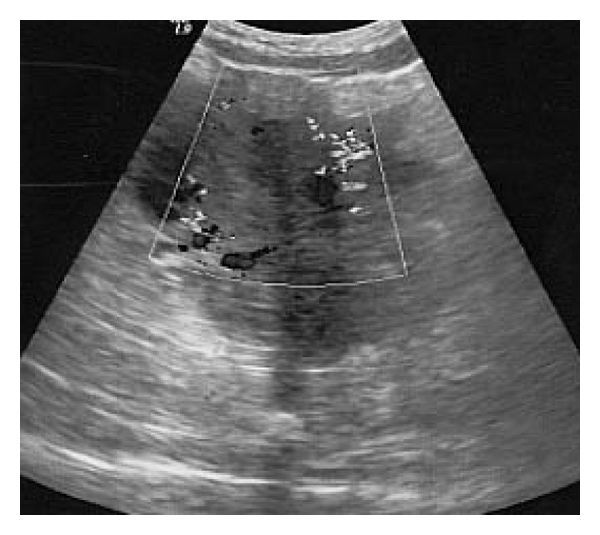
Color-Doppler evaluation shows the decreasing of the periuterine Varices.
